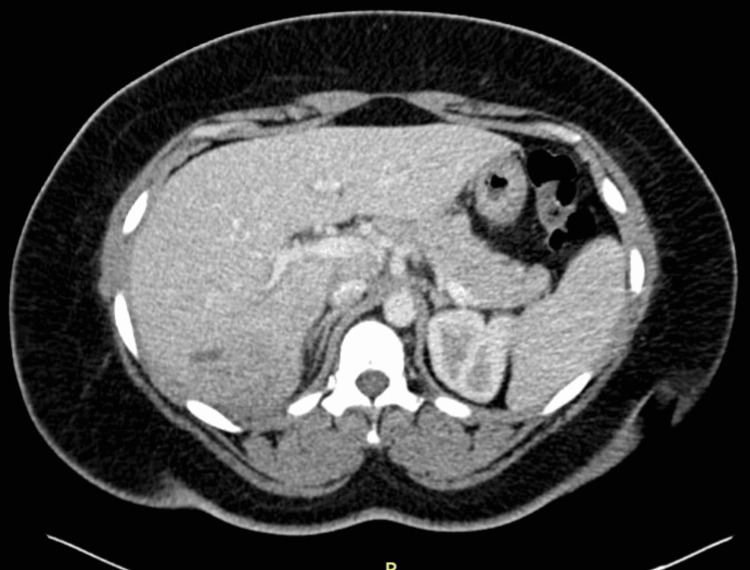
CT showing markedly abnormal appearance of the liver.
